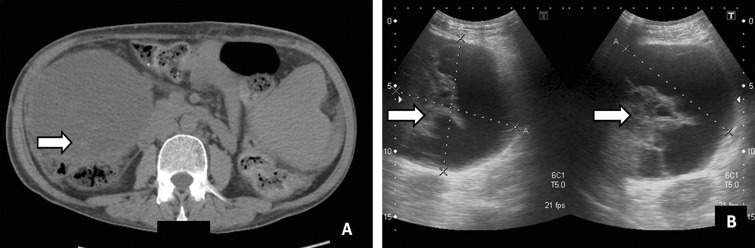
Hematoma. (A) Axial non-contrast CT scan image showing collection in the subhepatic location with hyperdense contents within (arrow). (B) Ultrasound image showing collection (arrows) with thick internal septations and internal echoes.
